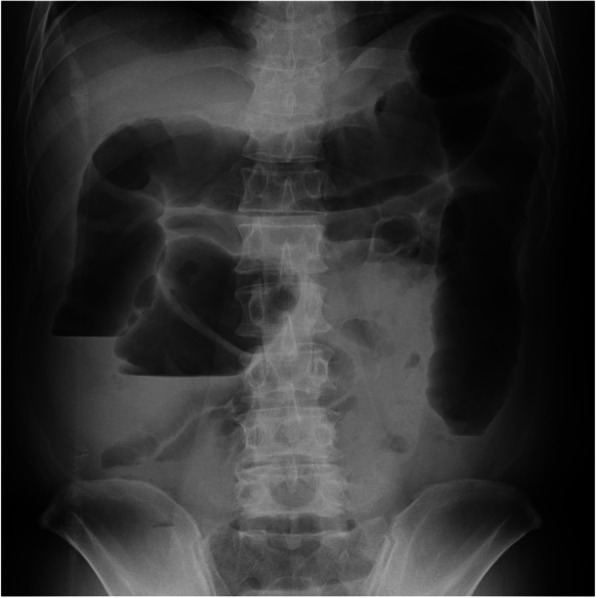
Abdominal X-ray. Megacolon is evident
